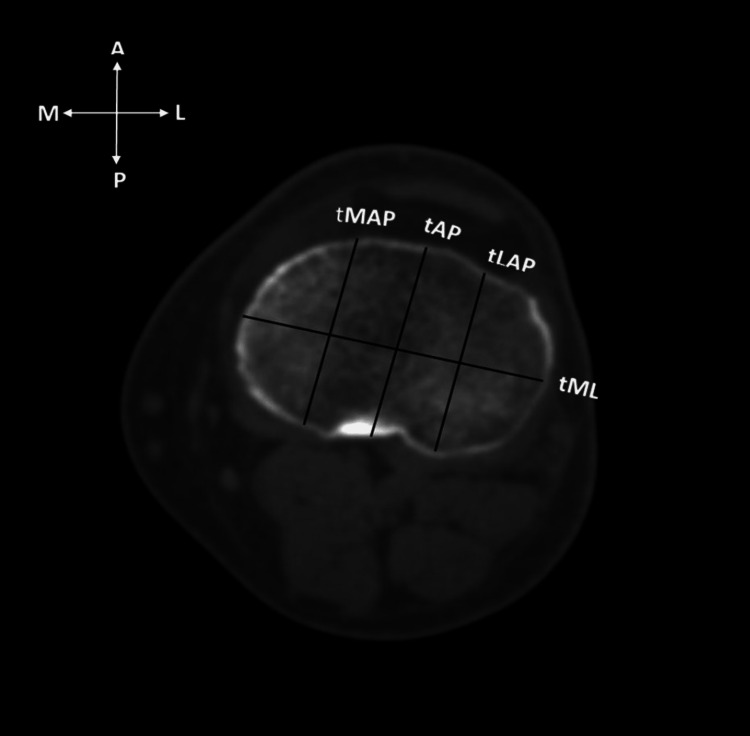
Axial CT image of the proximal tibia showing mediolateral length (tML), lateral anteroposterior length (tLAP), anteroposterior length (tAP), and medial anteroposterior length (tMAP). CT: computed tomography; tML: proximal tibial mediolateral length: tLAP: lateral anteroposterior length; tAP: anteroposterior length; tMAP: medial anteroposterior length.
